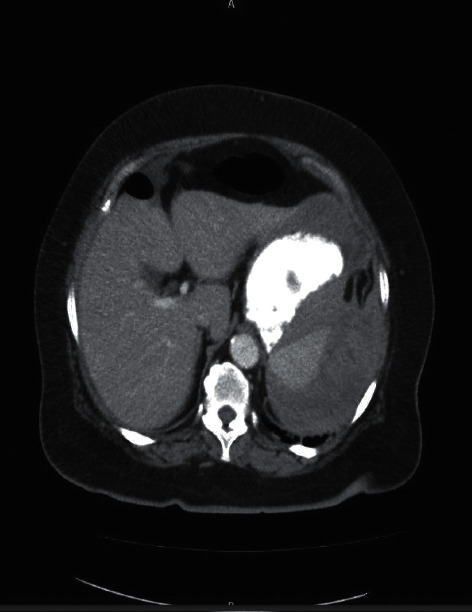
Axial CT scan, cross-section view, showing subcapsular hematoma of the spleen.
